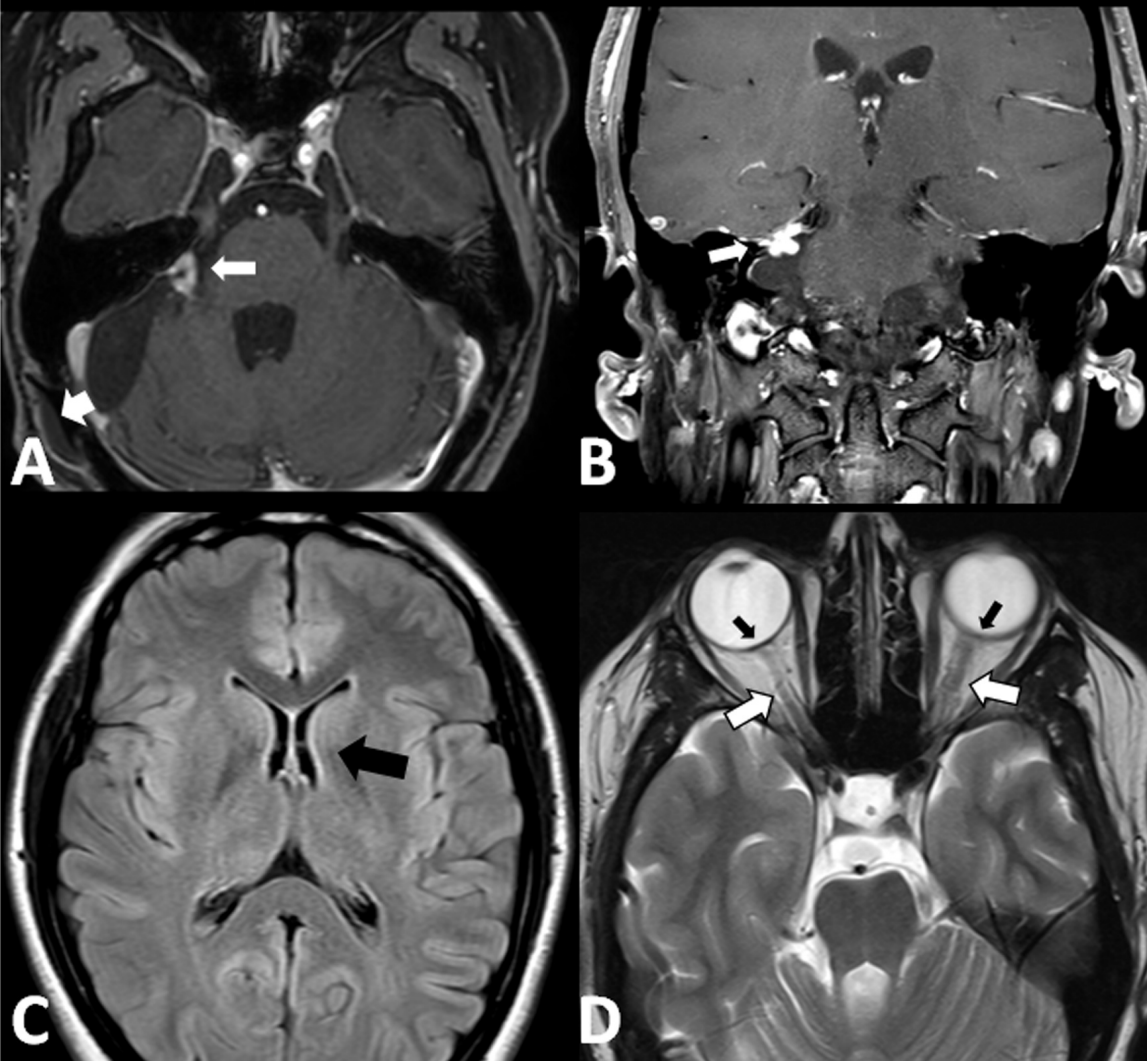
Magnetic resonance imaging (MRI) at two-year follow-up MRI performed two years after resection. (A) Axial T1-weighted gadolinium-enhanced image showing a 10 × 10 mm “fingernail” residual of the vestibular schwannoma (arrow) and persistent pseudomeningocele (arrowhead). (B) Coronal T1-weighted gadolinium-enhanced MRI. The residual is seen (white arrow). (C) Axial T2-weighted fluid-attenuated inversion recovery (FLAIR) image showing normal ventricle size. (D) Axial T2-weighted image shows marked bilateral reduction in the dilatation of the subarachnoid space within the dural sleeve surrounding the optic nerves (white arrows) and normal curvature of the globes (black arrows).
